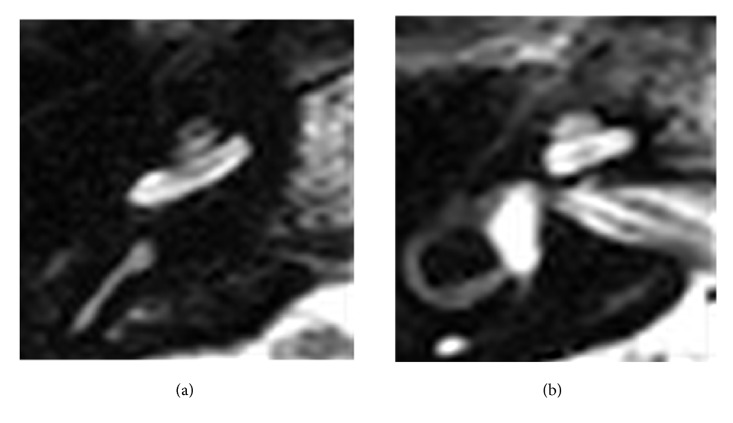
(a) Regular double-signal scalar in a T2-weighted MRI sequence (basal turn). (b) Regular double-signal scalar in a T2-weighted MRI sequence (second turn).
